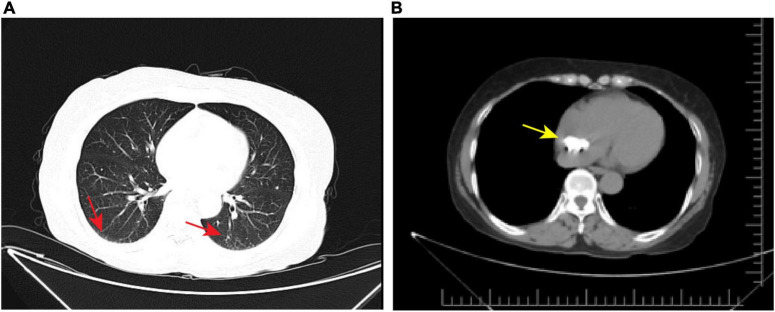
Axial view of chest computed tomography. (A) Chest CT showed low levels of inflammation in both lungs (red arrow). (B) Chest CT also showed a dense patchy shadow in the RA (yellow arrow).
